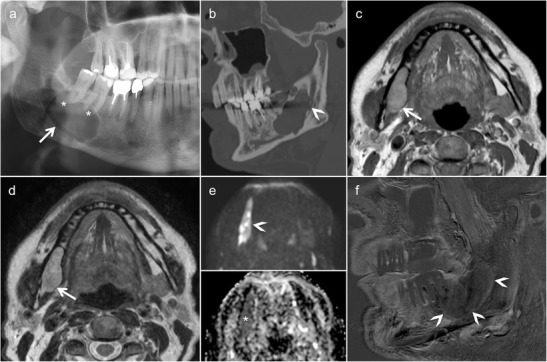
Characteristic radiological findings of odontogenic keratocyst (OKC) as seen in an elderly male. a OPT shows a large, well-defined osteolytic lesion involving the body and angle of the mandible on the right encompassing the right inferior alveolar nerve canal (*arrow*). Absence of root resorption (*asterisks*). b Multiplanar reformatted CT image (bone window) shows an unilocular cystic expansile lesion extending into the right inferior alveolar nerve canal (*arrowhead*). No periosteal reaction or pathological fracture. T1 (c) and T2 (d) axial images reveal thinned cortex (*arrows*) with postero-inferior cortical breach. e DWI reveals high signal on the b = 1000 image (*arrowhead*, *upper image part*) and low signal on the ADC map (*asterisk*, *lower image part*, ADC = 0.7 × 10^−3^ mm^2^/s), compatible with restricted diffusion due to intralesional ortho-/parakeratin accumulation and/or haemorrhage. f Subtraction image (T1 post-contrast and T1 pre-contrast) in the sagittal oblique plane shows only a thin enhancing lesion rim (*arrowheads*)
